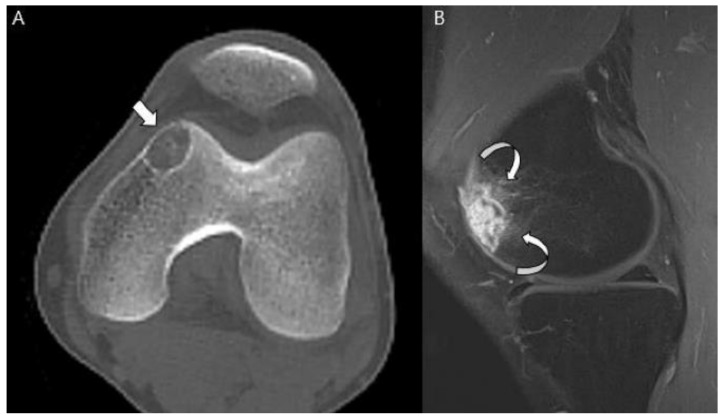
Clear cell chondrosarcoma of the distal femur in a 31-year-old man. (A) Axial CT scan shows an osteolytic lesion with a thin sclerotic margin at the distal femur (arrow). (B) Sagittal T1-weighted enhanced image with fat suppression shows a heterogeneously enhancing lesion with mild peritumoral enhancement at the distal femoral epiphysis (curved arrows).
